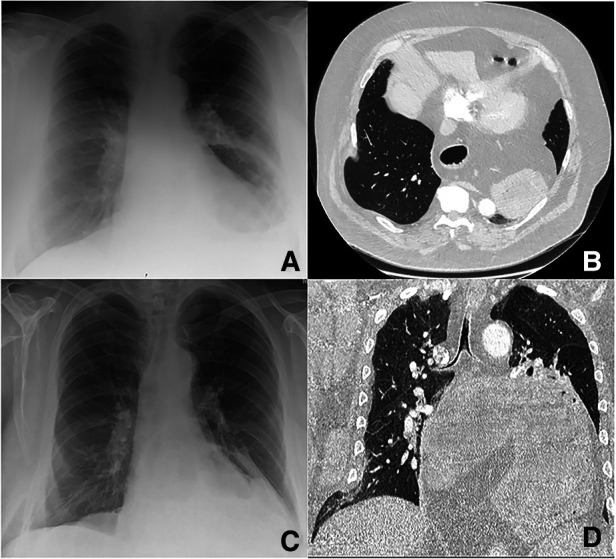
67-year-old man of BMI 42.5 who failed SLIH conservative management: the diagnostic and follow-up radiological pathway is shown. At first presentation, a chest x-ray (A) and CT abdomen were done to investigate for abdominal and lumbar pain: the defect was not evident at the x-ray and not described on the CT report (though being visible at the scan images). A large known hiatus hernia was reported at CT containing almost the entire stomach (with no signs of obstruction/strangulation) and the midportion of the body of pancreas. The patient presented again after 8 days with a second episode of acute pain and was admitted in the hospital: a new chest x-ray and CTPA (B) recorded an atelectasis of the lingula and suspicion for infection/aspiration. The herniation of the right lower lobe was still not visible at the x-ray, but it was characterized at the CTPA (B) and addressed as the possible cause of pain in the report. At repeat radiological evaluation for the new onset of shortness of breath 3 weeks after first presentation, the herniation of the right lower lobe was partially visible at the x-ray (C). The CTPA described an acute fracture of the right lateral eighth rib with associated lung contusion and atelectasis and small focal anterior right pneumothorax (D).
